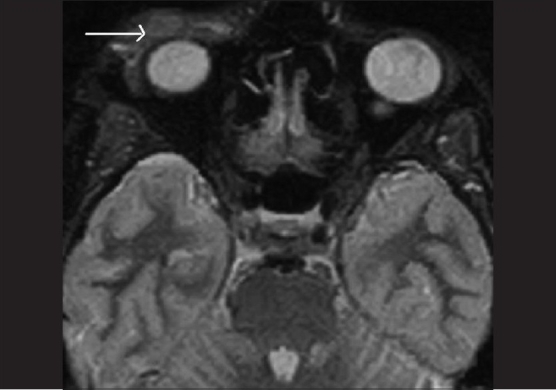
MRI fat suppressed STIR sequence showing the lesion
